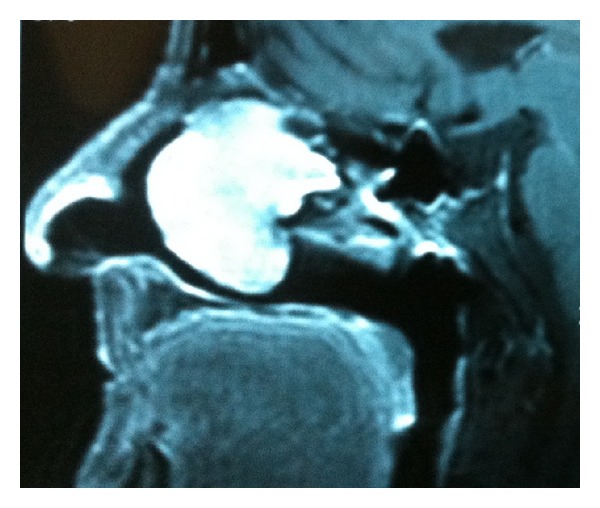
Sagittal MRI view of glomangiopericytoma extending along the olfactory fissure area.
